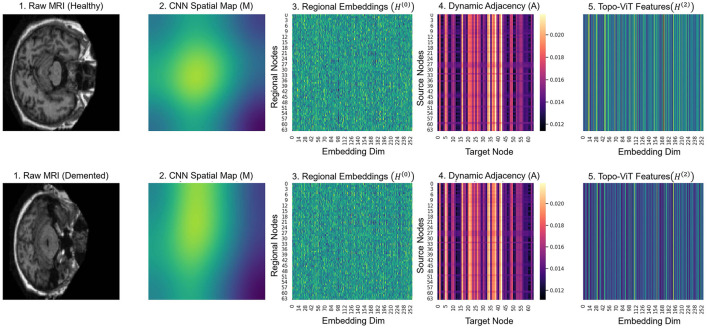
Hierarchical feature transformations from raw structural MRI to topology-aware global representations for healthy and demented samples.
